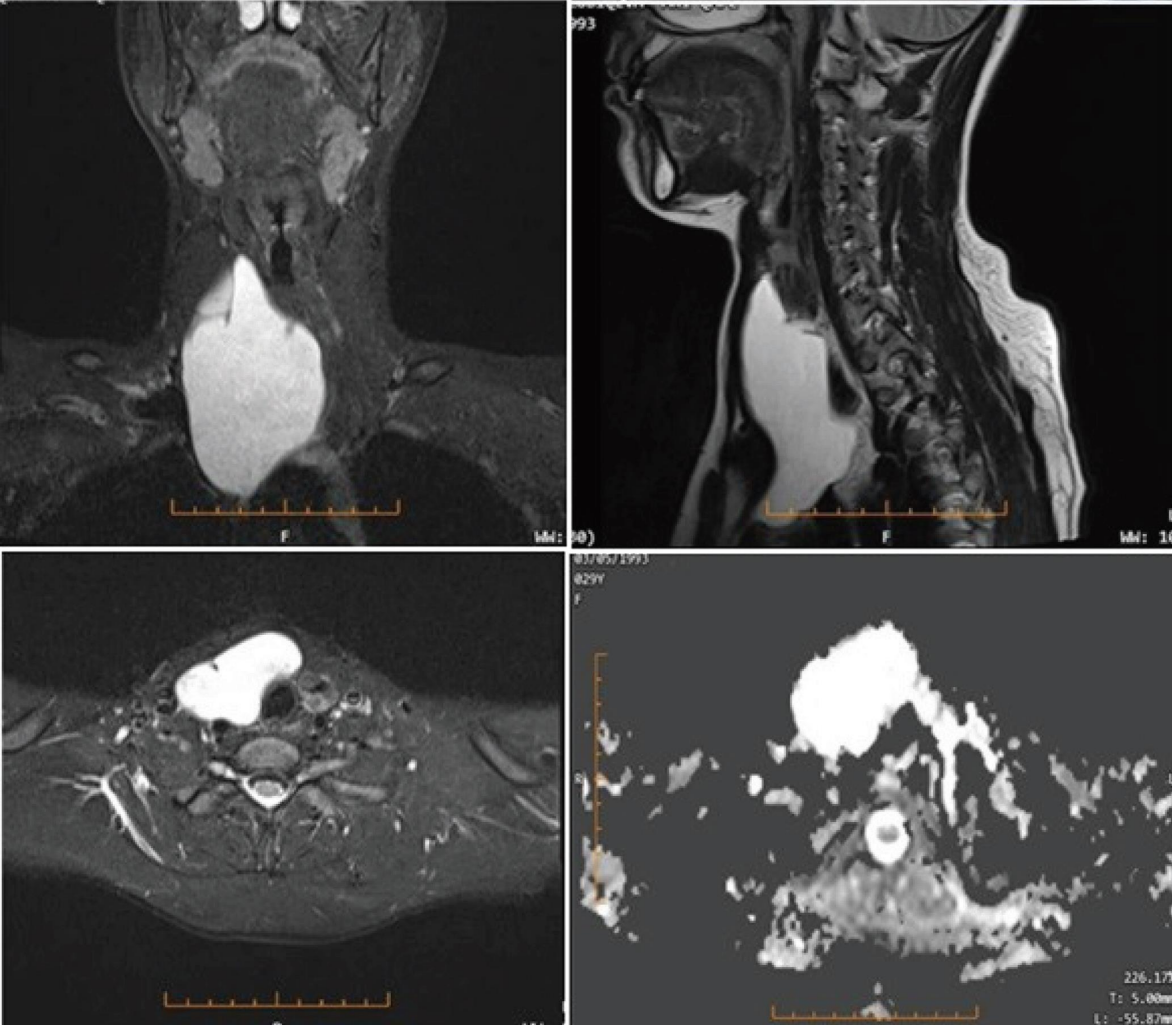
Magnetic resonance imaging (MRI) showing a well‐circumscribed, septated, T2‐hyperintense cystic lesion in the anterior right cervical region with extension into the upper anterior mediastinum. The lesion displaced the right thyroid lobe posteriorly and caused mild tracheal deviation without stenosis. It measured approximately 3.8 cm × 7 cm × 10 cm, contained proteinaceous fluid and showed no contrast enhancement.
